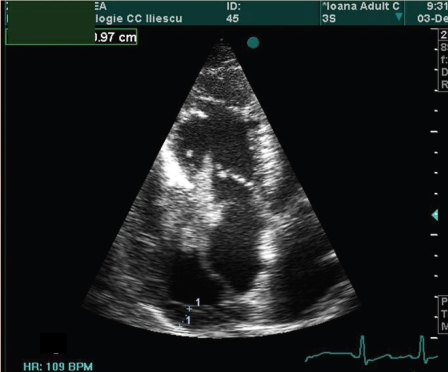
The same apical four–chamber plan. Ventricular systole. The tumor is inside the right ventricular cavity passing through the semiclosed tricuspid cusps.
